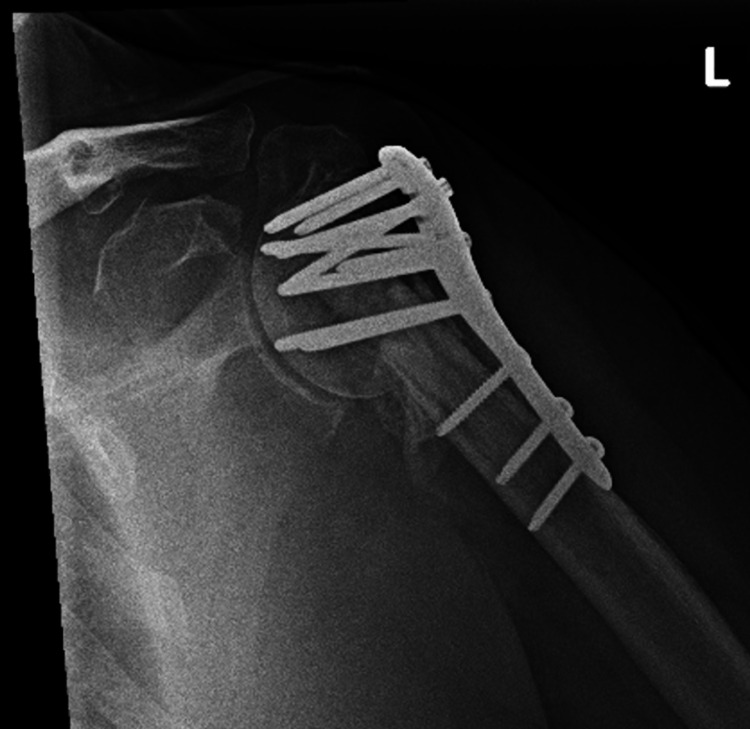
Anteroposterior (AP) radiograph of the left shoulder with varus collapse and failure of fibular strut augmentation.
